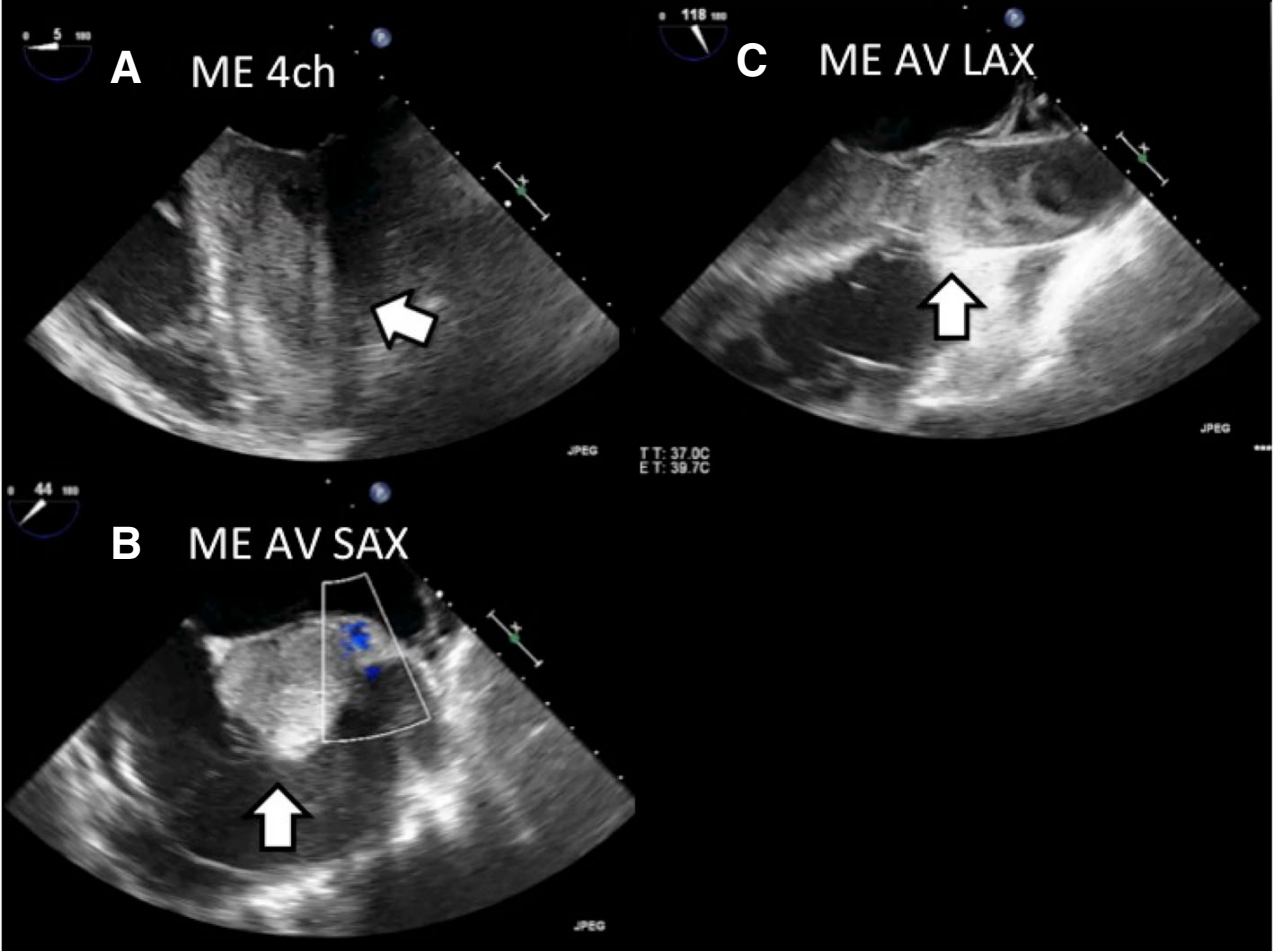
Transesophageal echocardiography (TEE) after the onset of atrioventricular block. a Mid-esophageal 4-chamber (ME 4ch) view showed thrombus formations and congestion in the left ventricle. b Mid-esophageal aortic valve short-axis (ME AV SAX) view showed thrombus formations in the Valsalva sinus and aortic valve closure. c Mid-esophageal long-axis (ME AV LAX) view showed thrombus formations in the left ventricular tract and the Valsalva sinus
